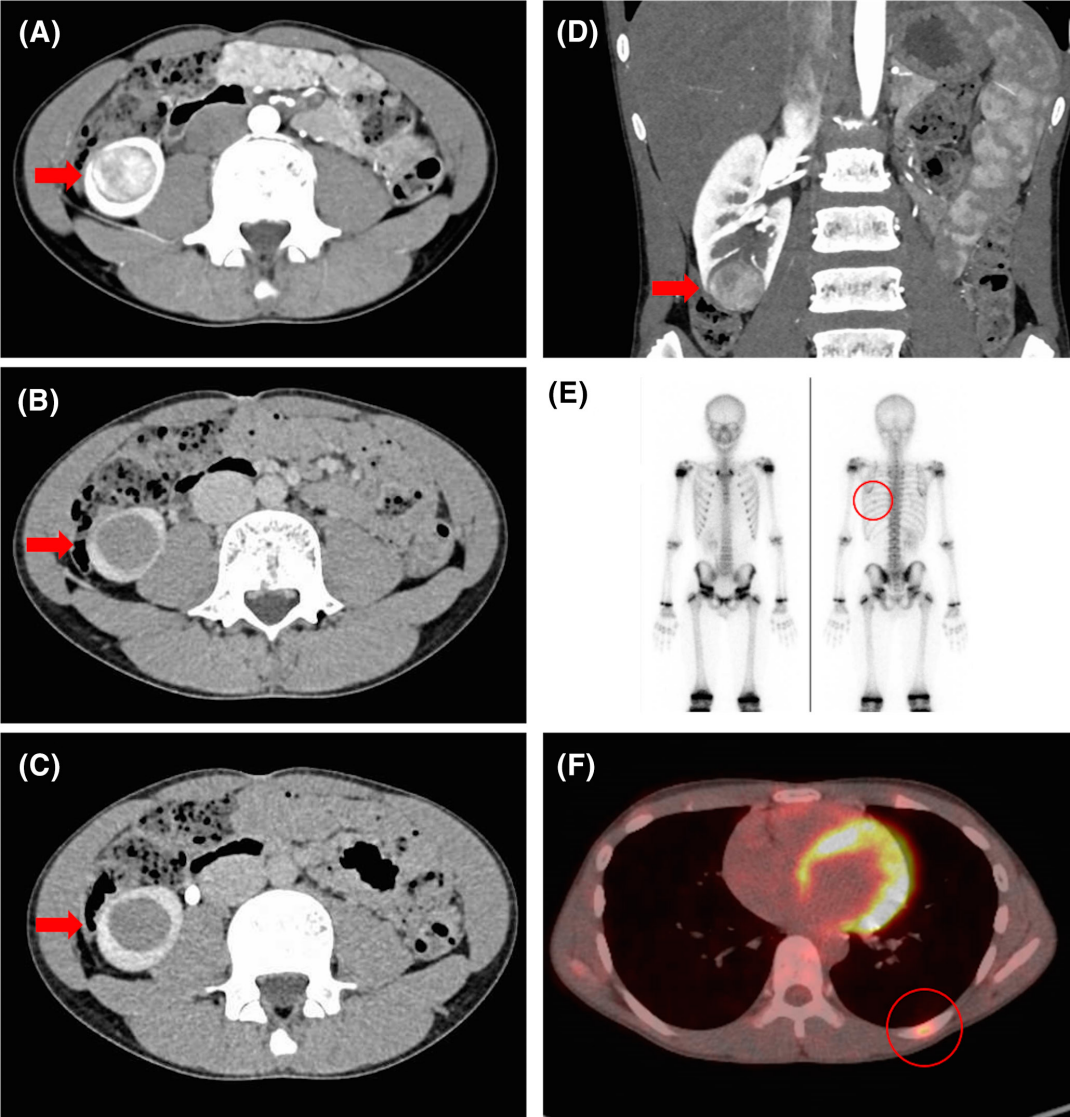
(A–D) Contrast‐enhanced CT showed a well‐defined 30 × 25 mm‐sized mass in the lower pole of the right kidney in the early phase, with a weak contrast effect below the renal cortex in the equilibrium phase and washout in the drainage phase (red arrow). (E, F) Bone scintigraphy and positron emission tomography‐CT showed an accumulation in the left eighth rib, which was suspected of metastasis (red circle).
